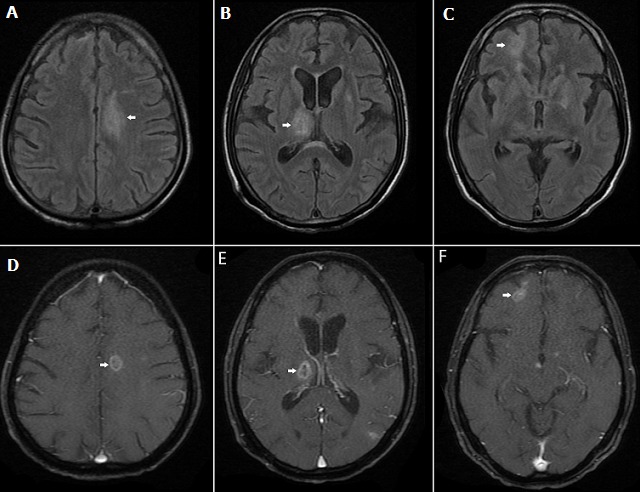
MRI Brain in CNS Toxoplasmosis
